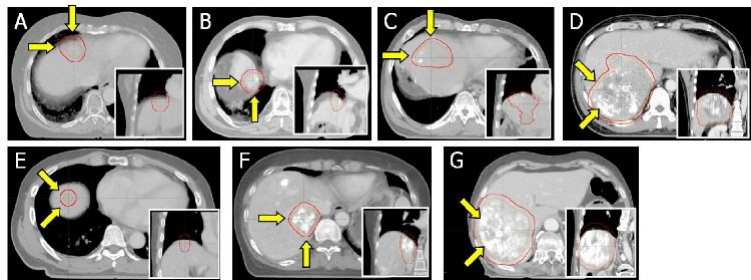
Axial views of the tumor centers on the AVG CT scans. The red contours are the PTV volume and the arrows indicate the entrance proton beam directions. The inset in each figure is the coronal view for tumor volume.
